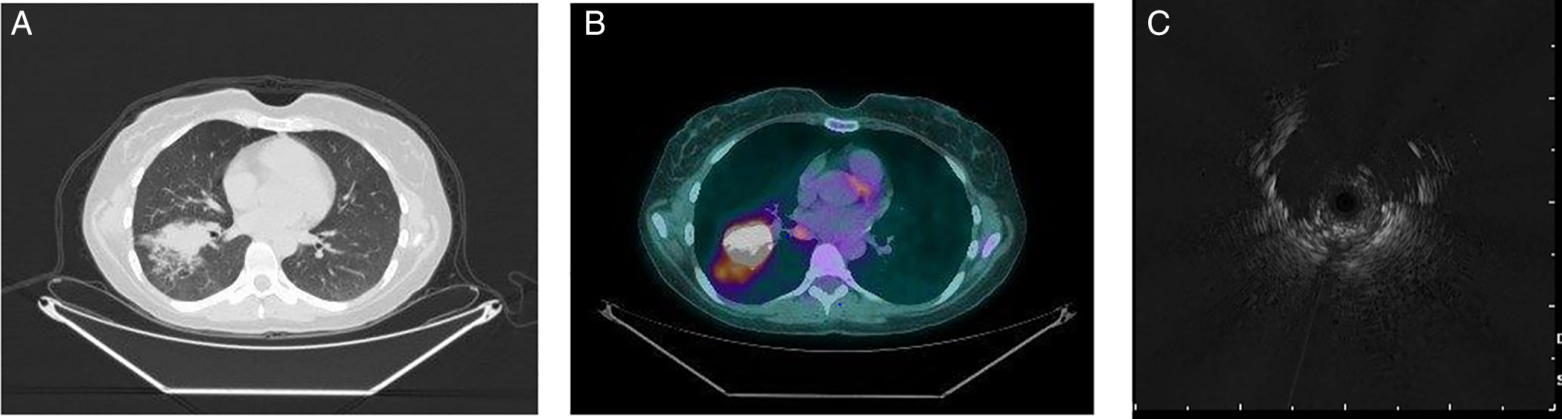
(A, B): Computed tomography (CT) chest showing right lower lobe opacity, 4.4 × 2.9 cm in axial dimension, positron emission tomography avid, and with a mass‐like appearance that was a cluster of nodules and micronodules earlier (17 × 11 mm in largest dimension). There are numerous surrounding micronodules and ground‐glass opacities. (C) Endobronchial radial probe ultrasound image of the lesion.
